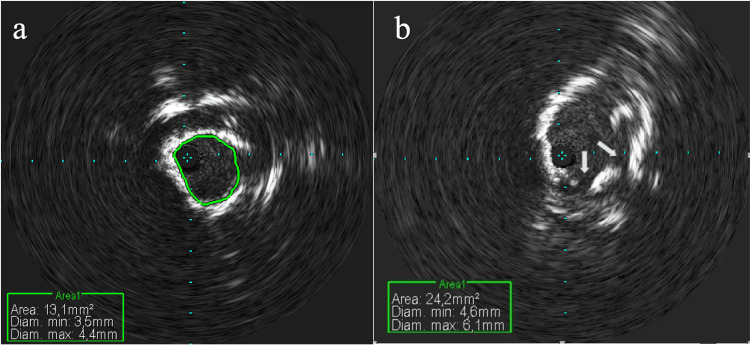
(a) Intravascular ultrasound, semi-concentric calcifications at the level of the stenosis; (b) after intravascular lithotripsy, intravascular ultrasound demonstrated calcium debulking (arrows) with an enlarged lumen area.
